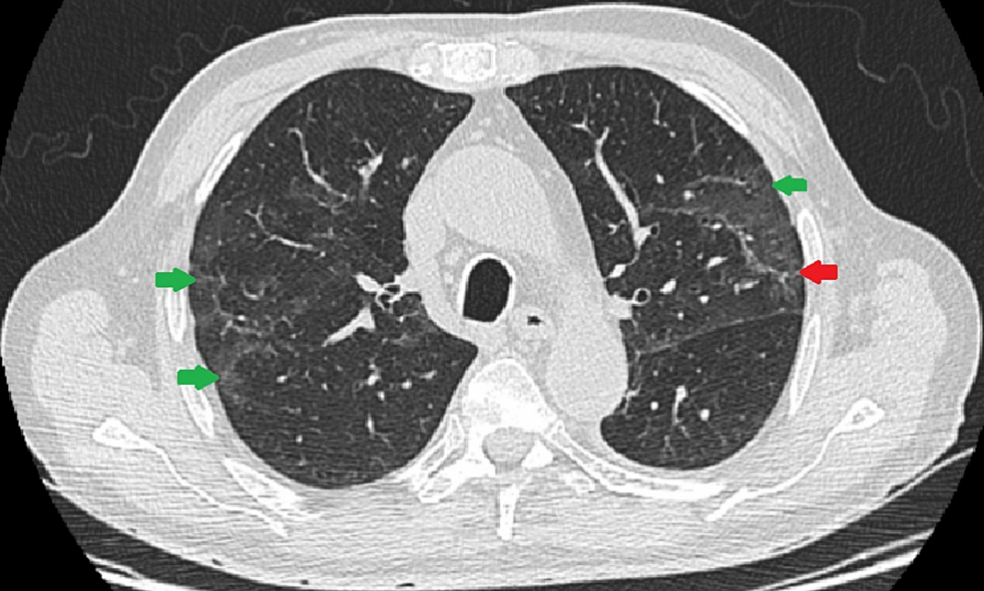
Thoracic CT scan performed in June 2021 Green arrows: fibrosis of the previously affected segments; red arrow: maintenance of some of the ground-glass infiltrates.
